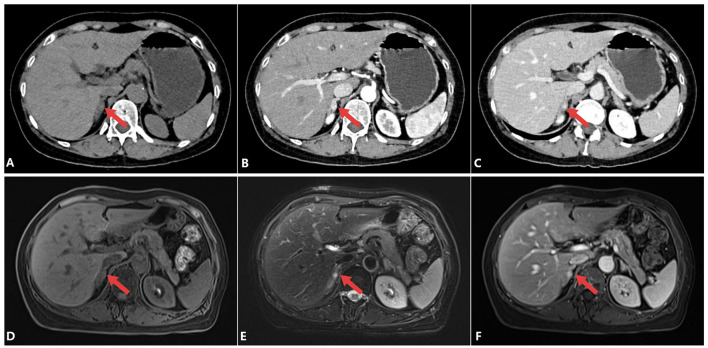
Contrast-enhanced abdominal CT (A–C) and contrast-enhanced adrenal MRI (D–F) revealed an enhancing right adrenal nodule highly suggestive of pheochromocytoma. Contrast-enhanced abdomen CT (A–C): (A) unenhanced, (B) arterial phase, (C) delay phase. Contrast-enhanced adrenal MRI (D–F) (D) T1 phase, (E) T2 phase, (F) enhanced phase.
